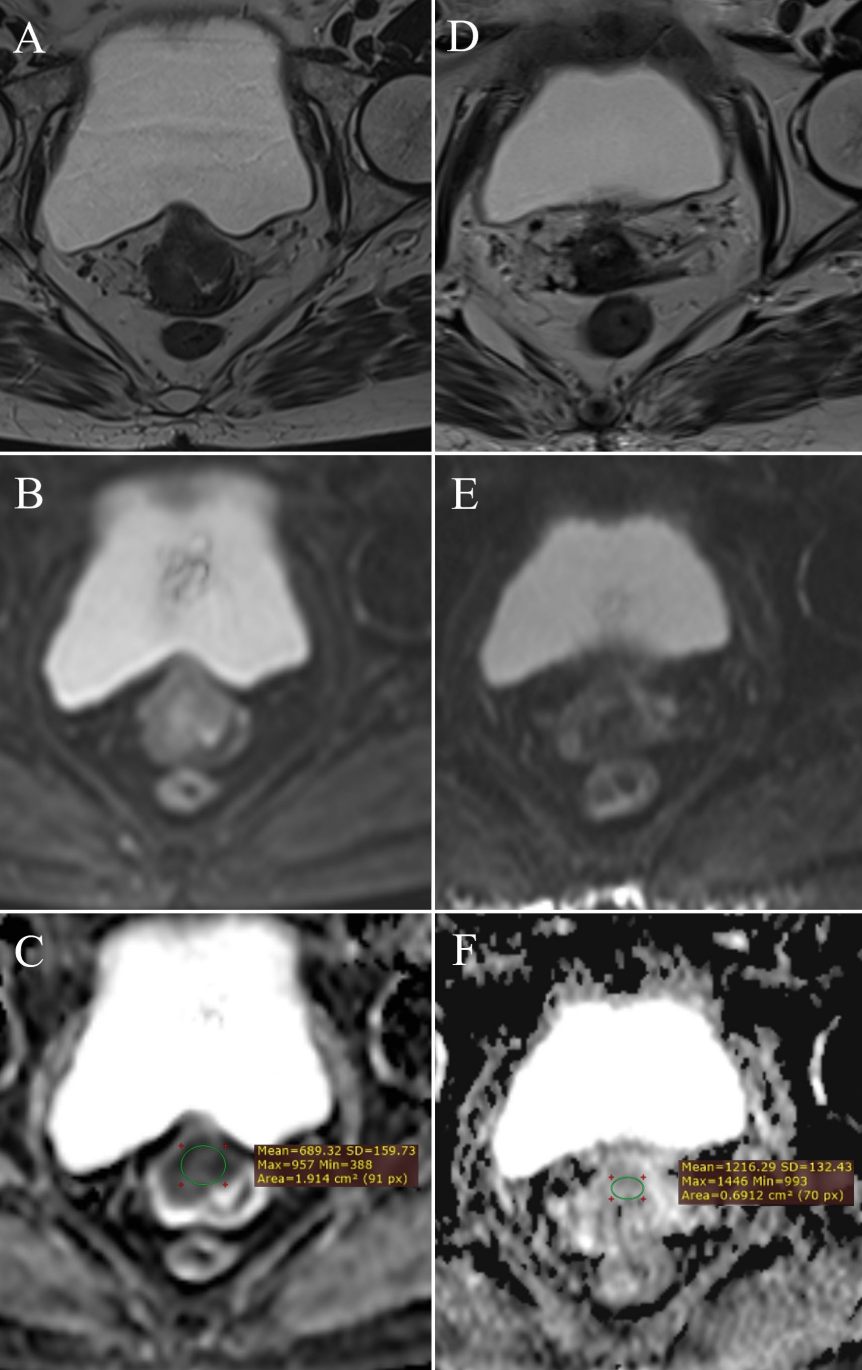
Change in ADC values: pre-therapy cervical cancer – (A) (T2W), (B) (DWI), (C) (ADC map with ROI); post-therapy cervical cancer – (D) (T2W), (E) (DWI), (F) (ADC map with ROI).
